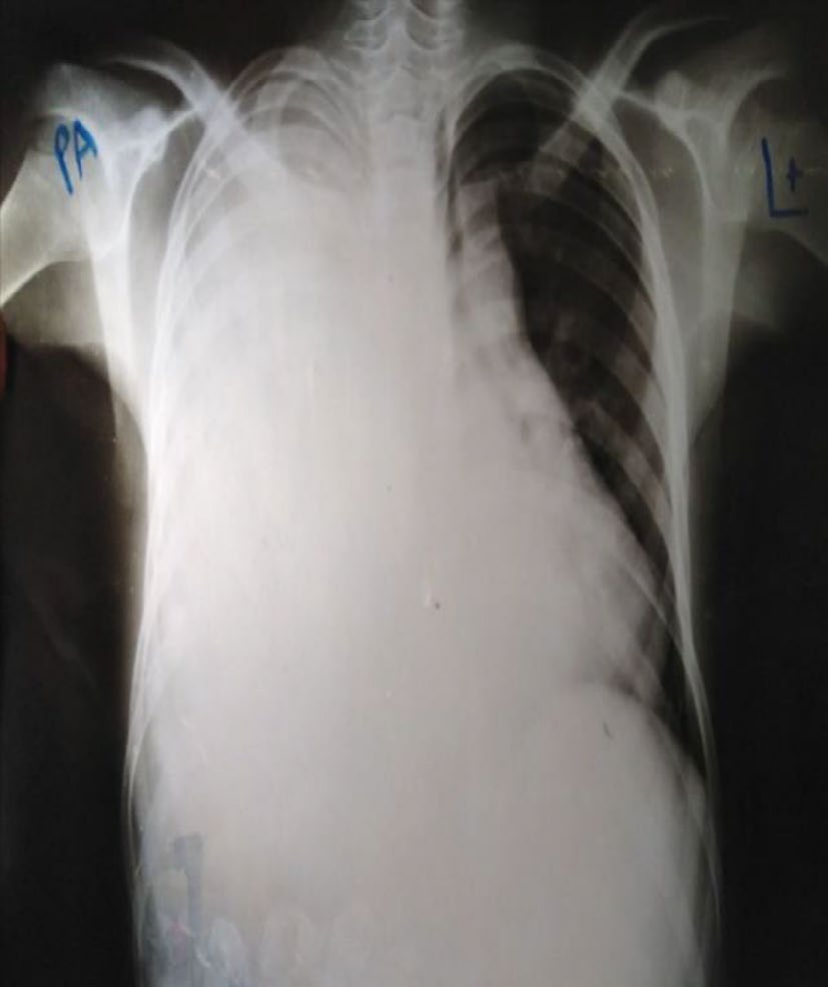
Poster‐anterior chest radiograph on admission (before the insertion of chest tube)
